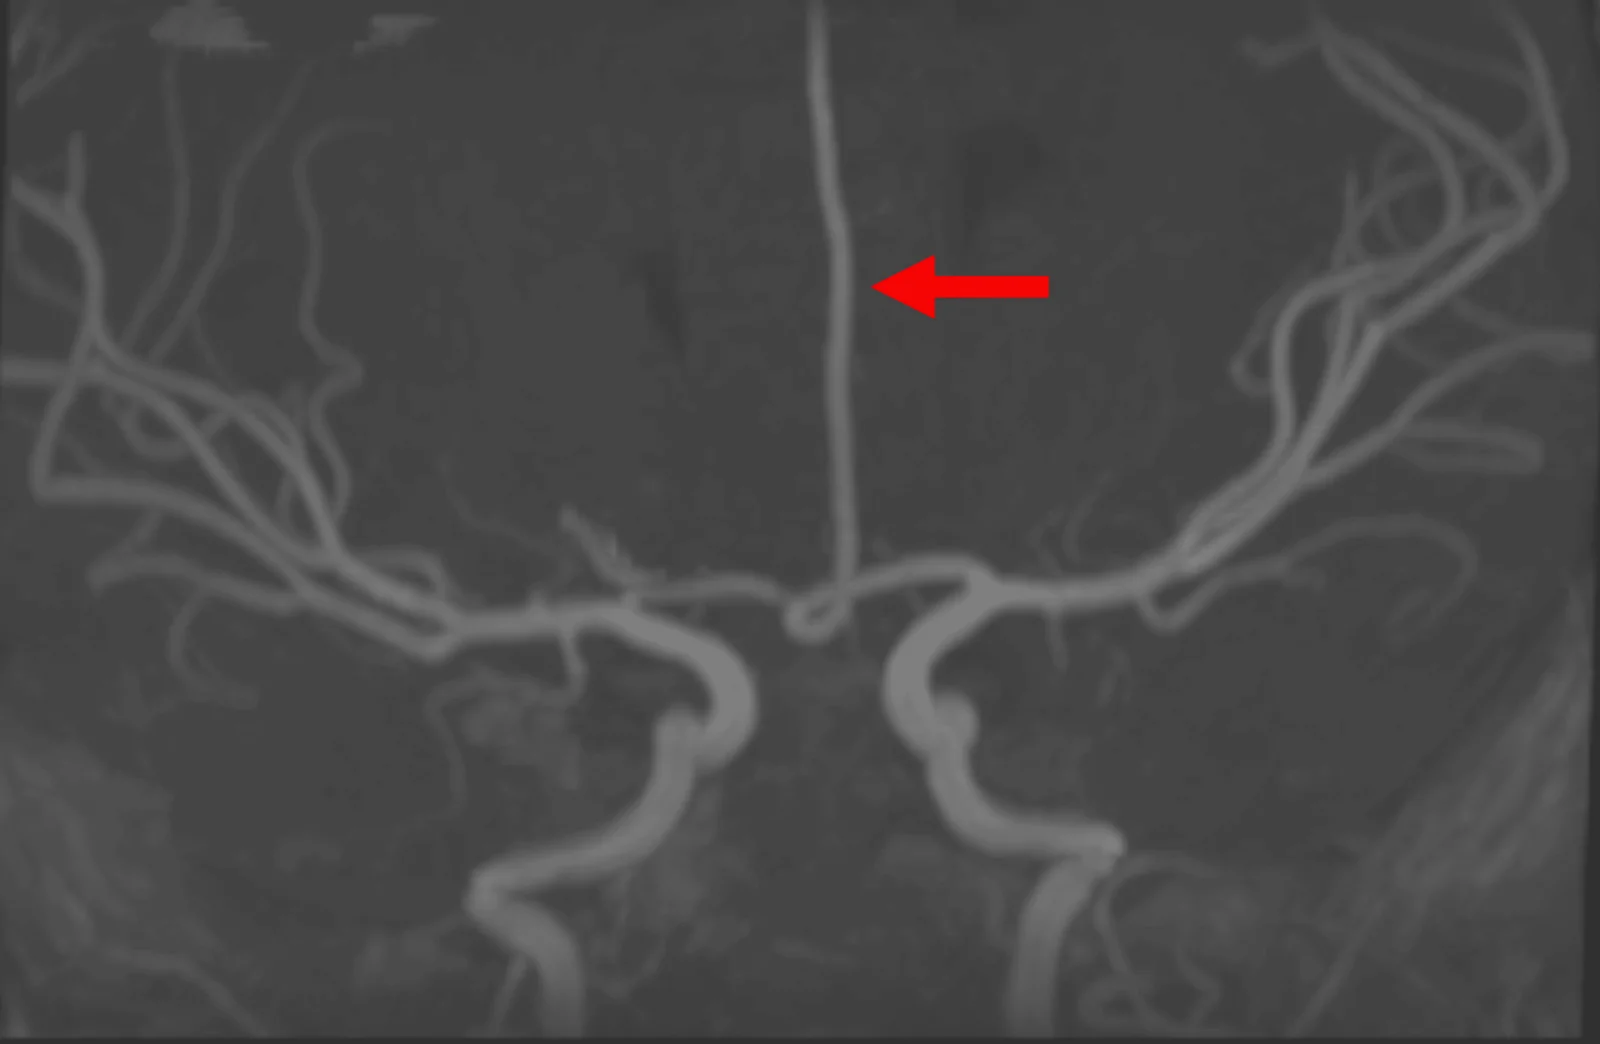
MRI image demonstrating an azygous anterior cerebral artery MR angiogram with 3D reconstruction shows an azygous anterior cerebral artery (red arrow).
